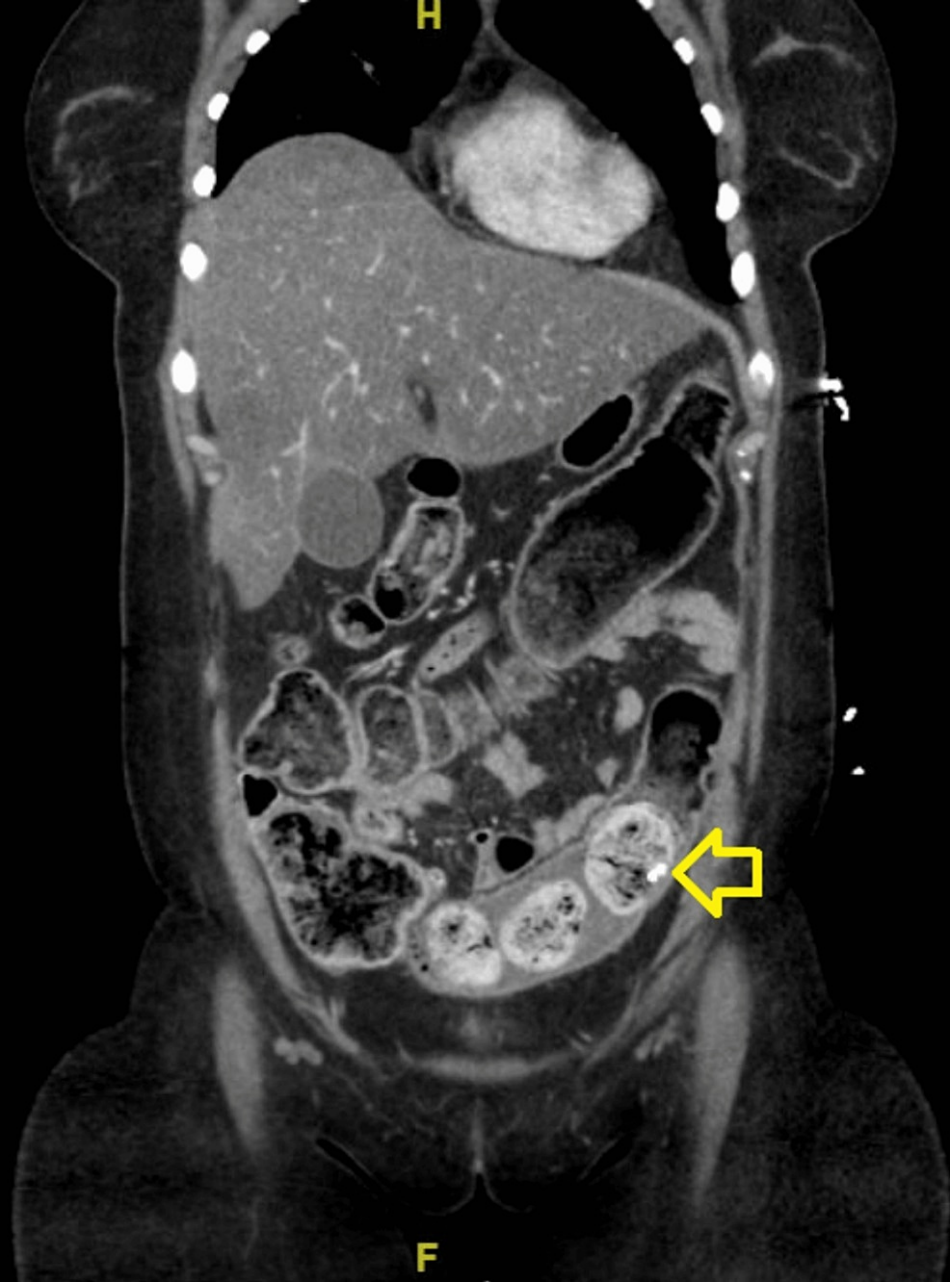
Coronal view computed tomography with intravenous contrast demonstrating a calcification in the sigmoid colon (indicated with yellow arrow)
